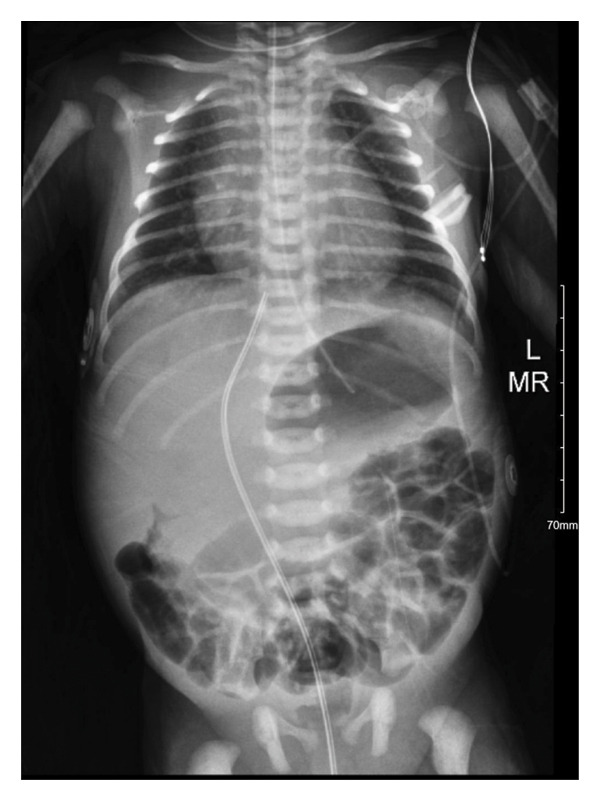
Supine chest–abdomen radiograph shows a markedly distended abdomen with relative paucity of bowel gas in the upper abdomen, most prominent near the hepatic margin, and inferior displacement of gas‐filled small‐bowel loops into the lower quadrants.
